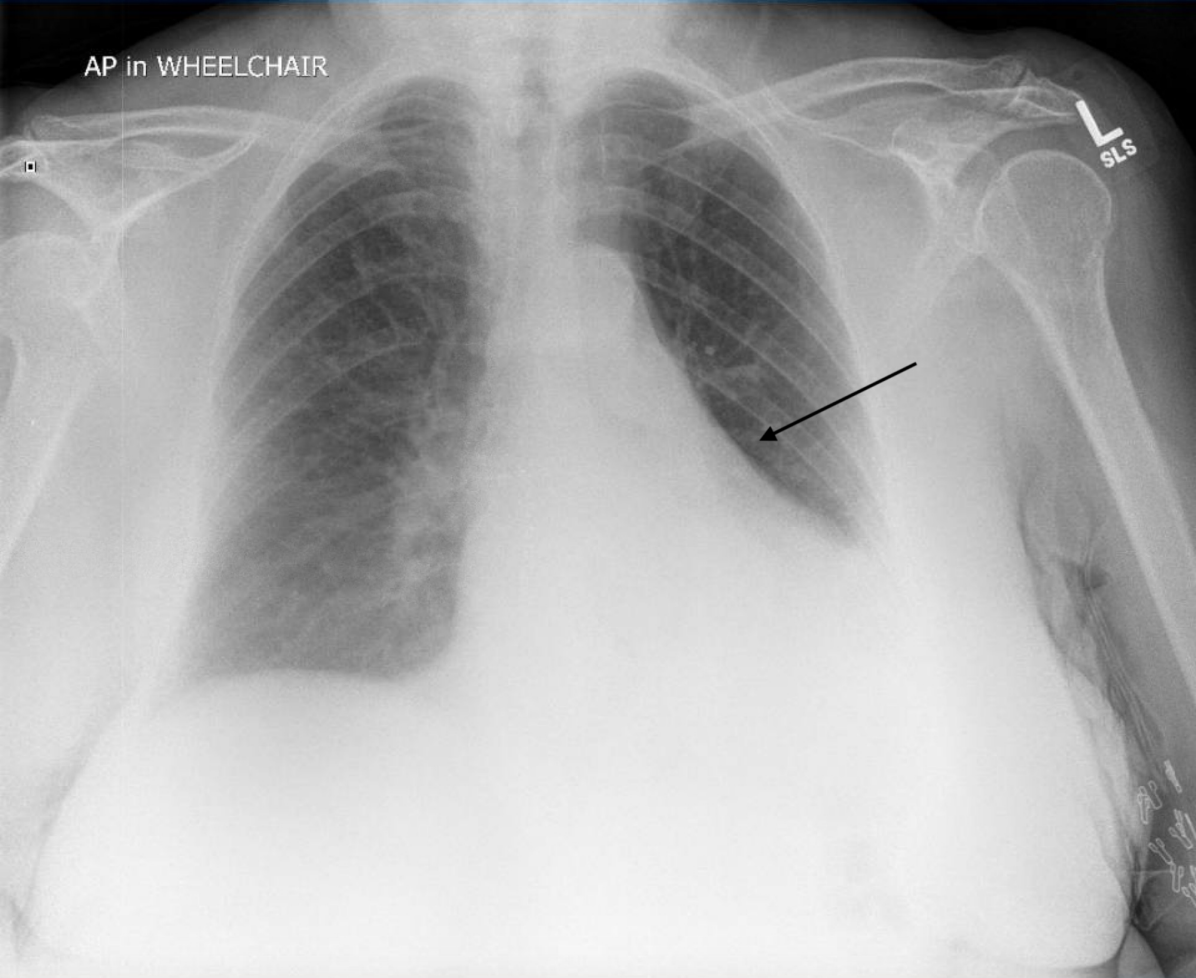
Left basilar consolidation seen on chest X-ray.
